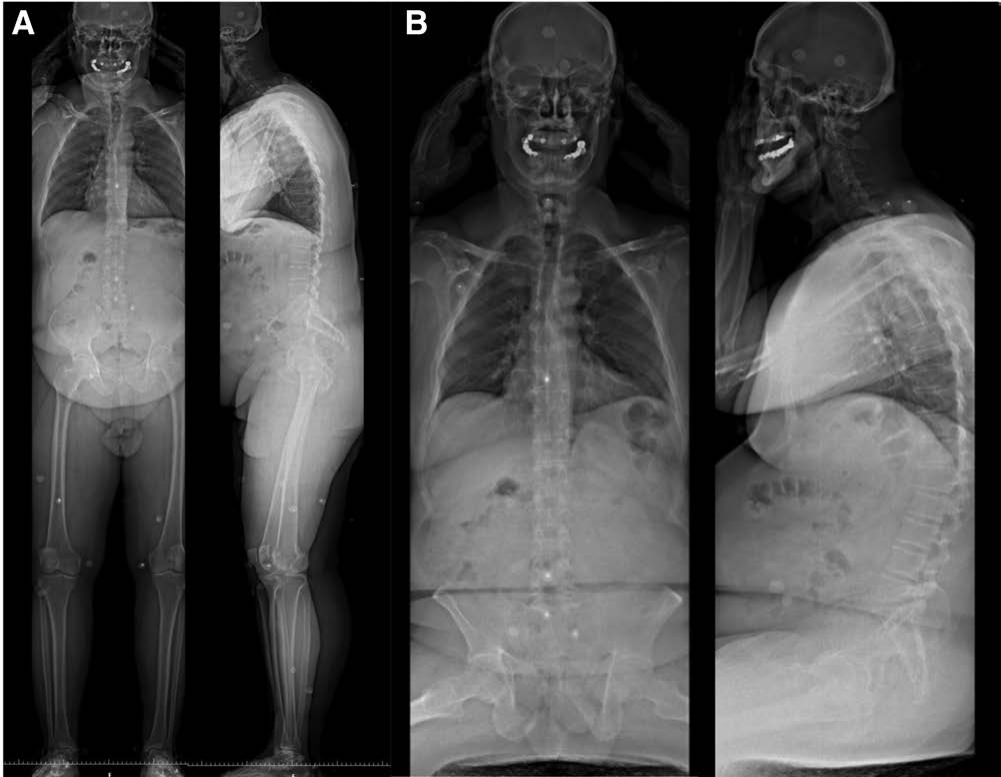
Baseline standing (A) and seated (B) full-body biplanar radiographs.
